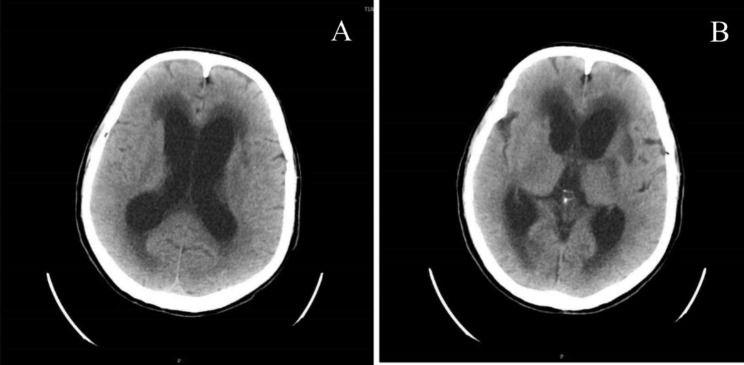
CT of the brain: dilatation of the supratentorial ventricular system and widening of the sulcioencephalic fissure
